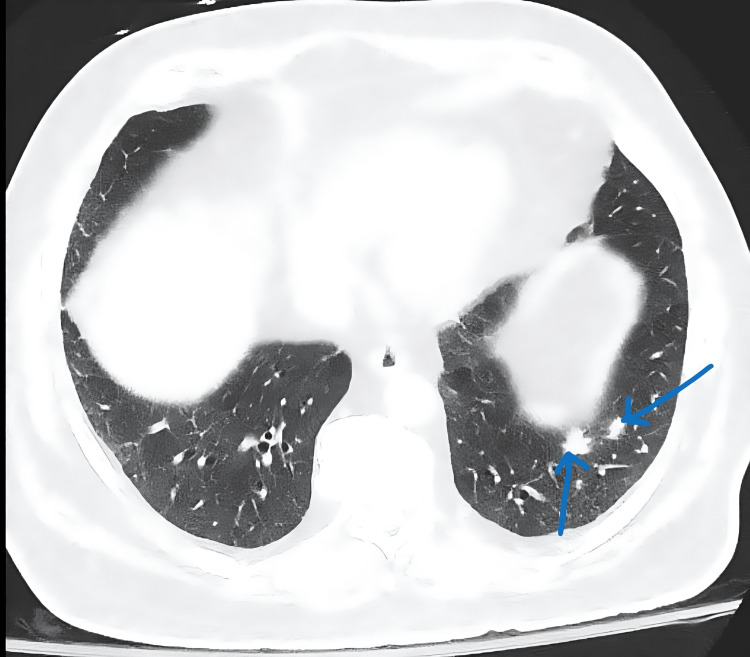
CT of the chest showing multiple pulmonary nodules suspicious for metastasis Arrows highlight representative metastatic nodules in the lung fields. CT: computed tomography
